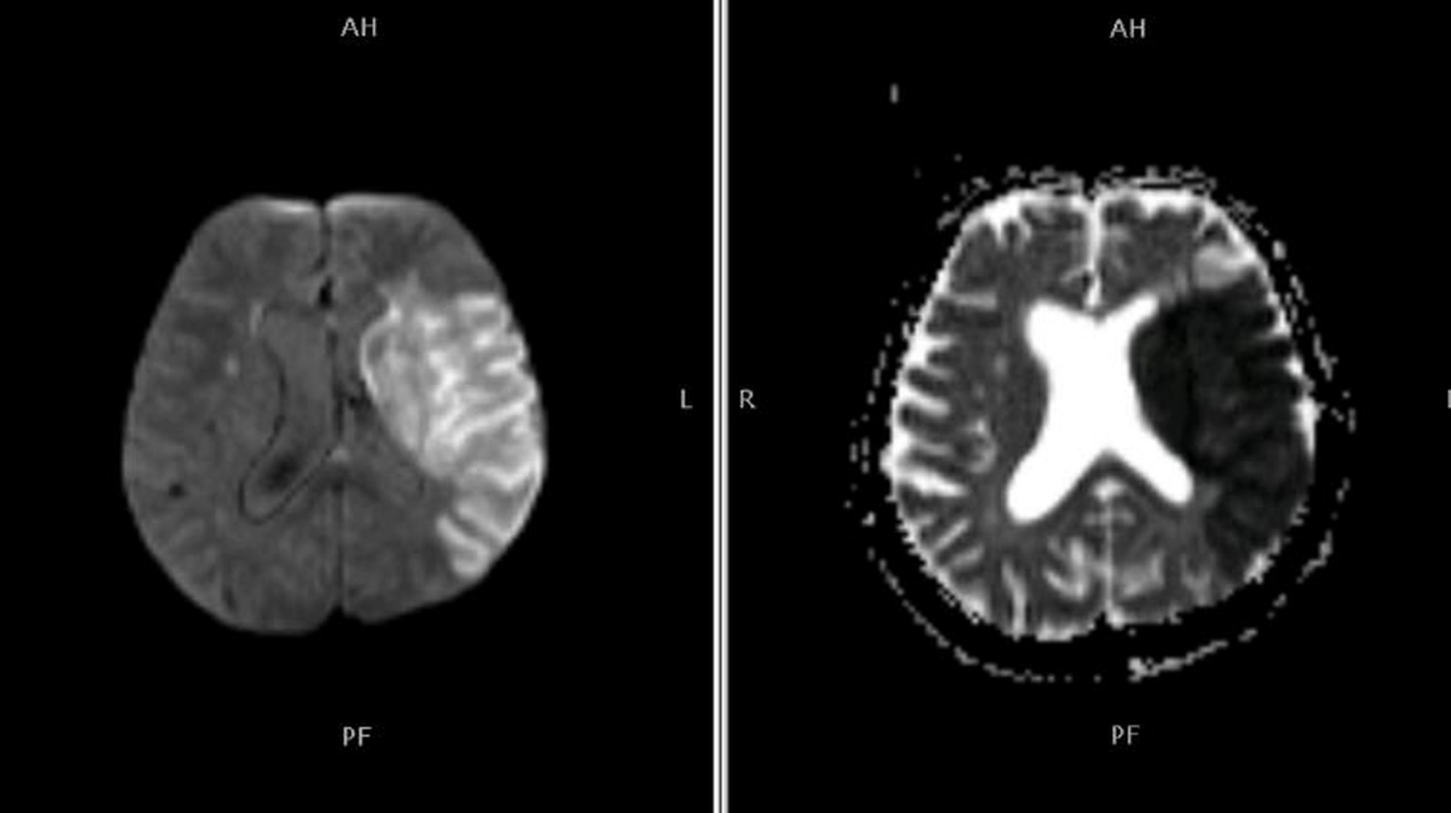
Territorial infarct pattern Diffusion-weighted imaging (DWI) shows bright DWI and low apparent diffusion coefficient (ADC) indicating acute territorial infarct. Patient had a significantly poor outcome and had diabetes as well as hypertension.
